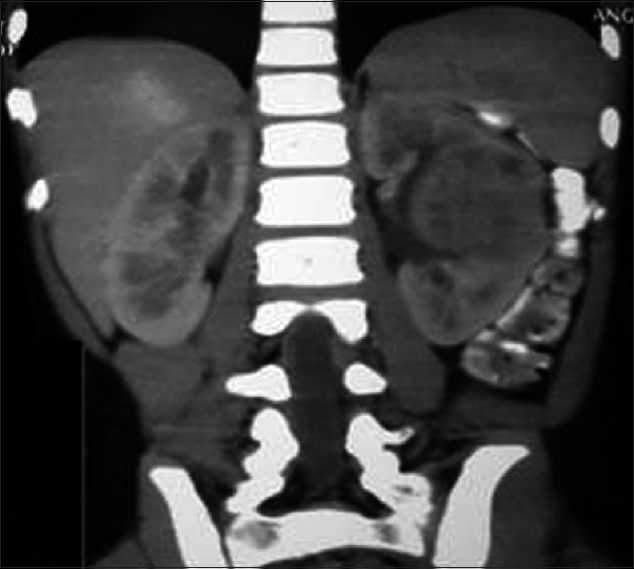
Contrast enhanced computed tomography picture of the lesion in the midpole of left kidney-along the column of bertini from papilla to cortex with calyceal effacement and poor enhancement of IV contrast
